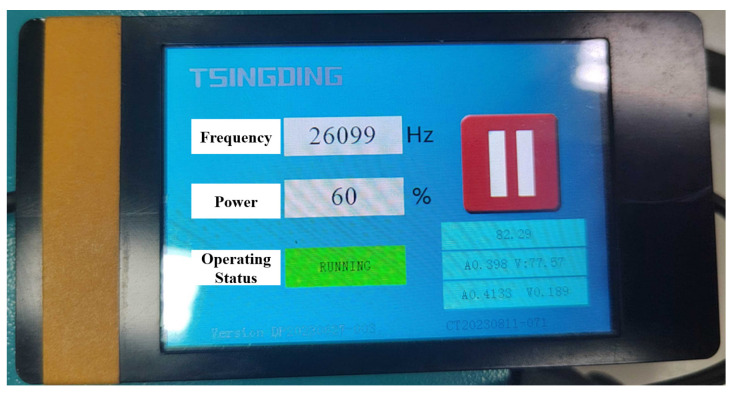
Ultrasonic vibration parameters.
